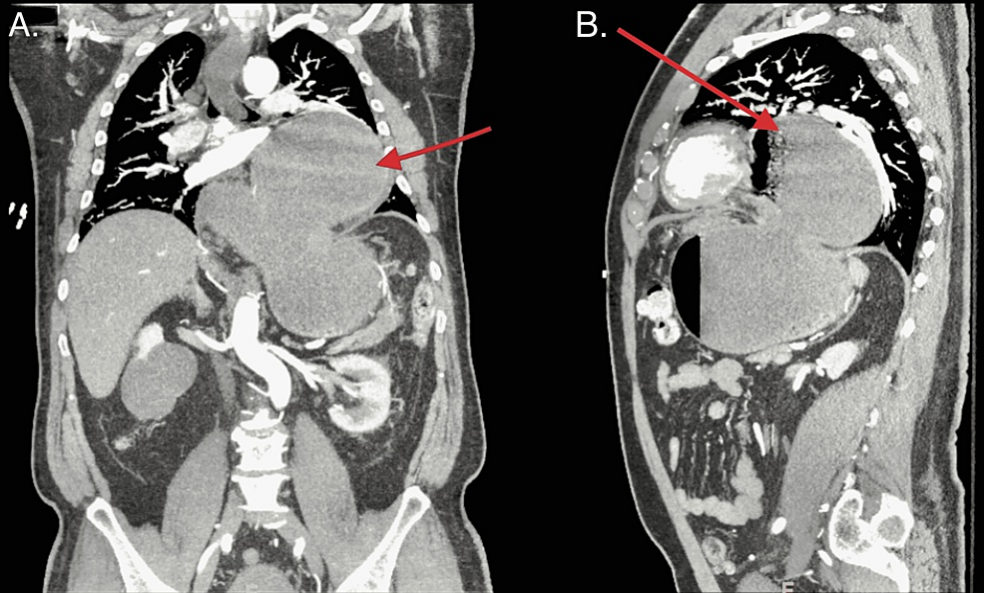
CT of the chest and abdomen demonstrating a large hiatal hernia (red arrow) and a dilated, fluid-filled esophagus.
